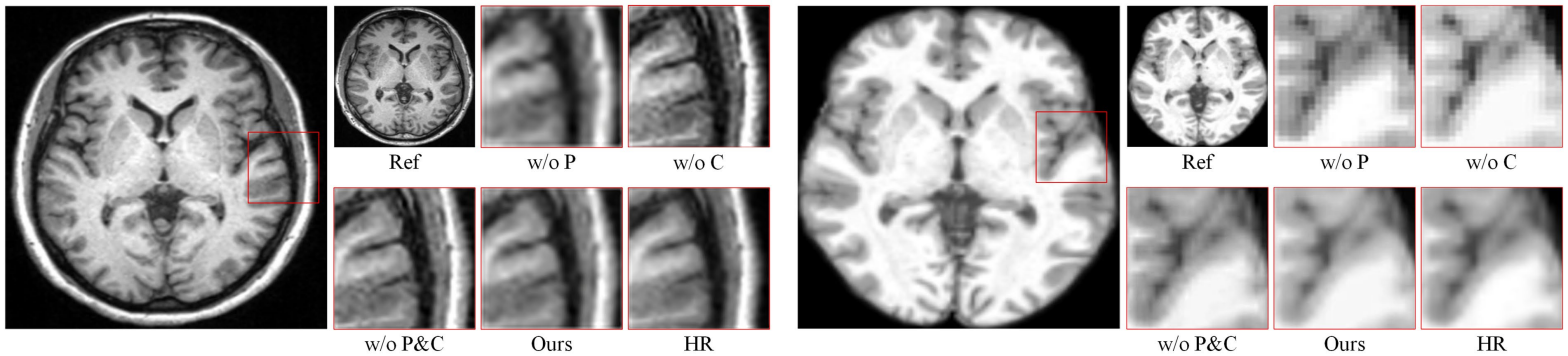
Visualization and ablation study of the index map (P) and score map (C) for reference-guided MRI super-resolution on the Brain (ours) dataset (×4). Two representative cases are shown, including the LR input, reference image, ablation variants, and zoomed-in regions for comparison.
